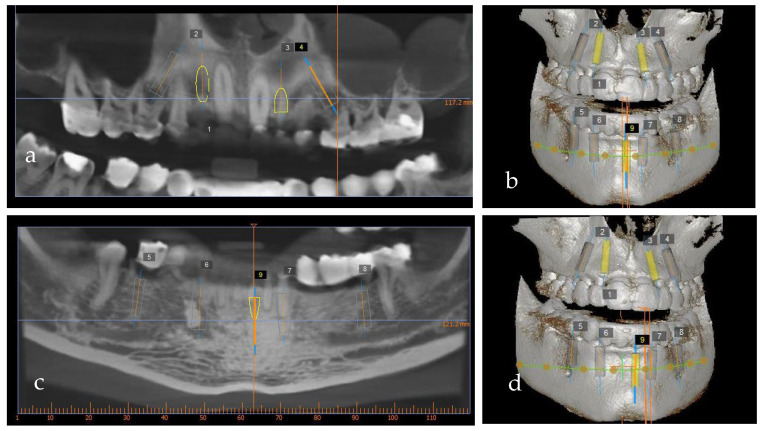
Implant planning software using cone-beam computerized tomography: (a,b) maxilla and (c,d) mandible.
